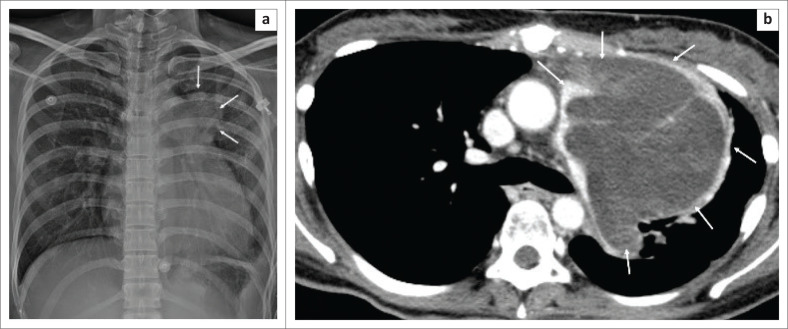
(a) Follow-up frontal chest radiograph revealed an enlarged main pulmonary artery and a left hilar mass (arrows). (b) Axial computed tomography pulmonary angiography demonstrated a large lobulated rim enhancing exophytic mediastinal mass extending from the dilated main pulmonary artery and the left pulmonary artery (arrows).
